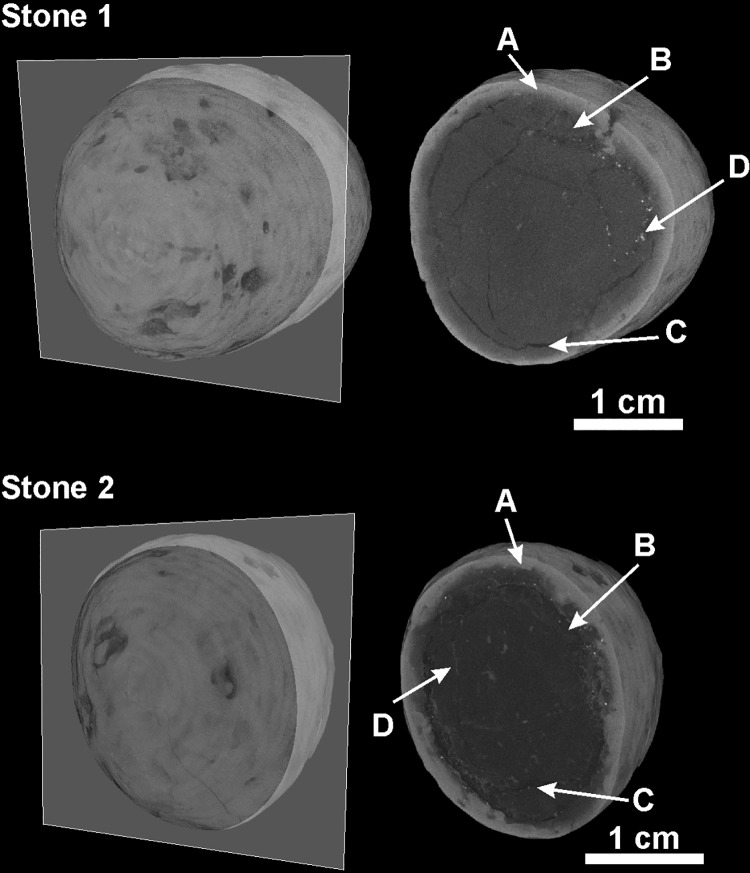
Internal structure. μ-CT reconstructed sections of stones S1 and S2: A: lighter grey colour, outermost portions; B: darker colour, innermost portions; C: black colour, fractures within the stone; D: light grey colour, calcite filling in some of the fractures.
